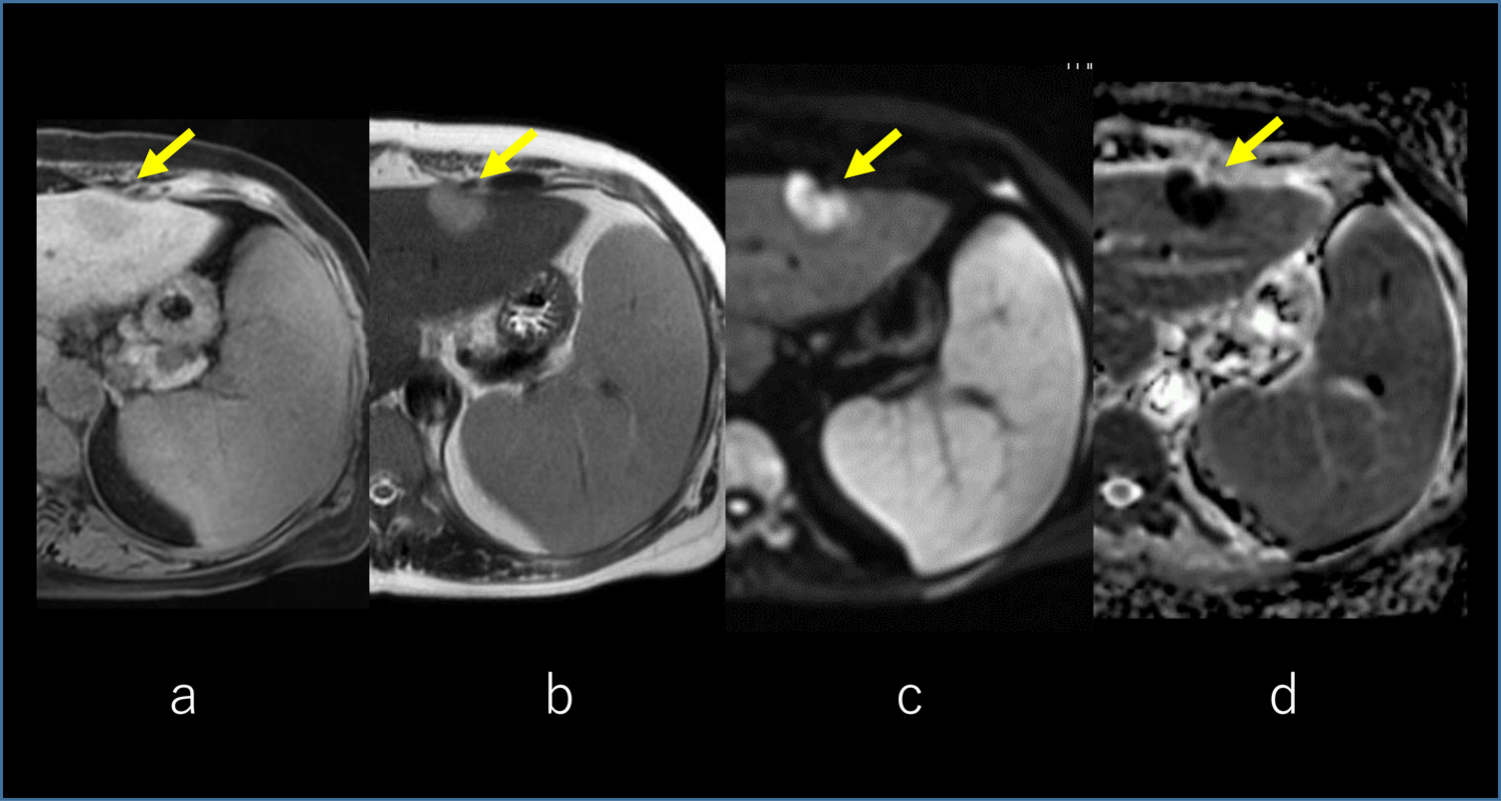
MRI of the liver. The tumor was visible in the left lobe which presents hypointense in T1-weighted image (arrow) (A) and slightly hyperintense in T2-weighted image (arrow) (B). The lesion was presenting with a high signal in the diffusion-weighted image (DWI) (arrow) (C) and a low signal in the apparent diffusion coefficient (ADC) map (arrow) (D). MRI, magnetic resonance imaging.
